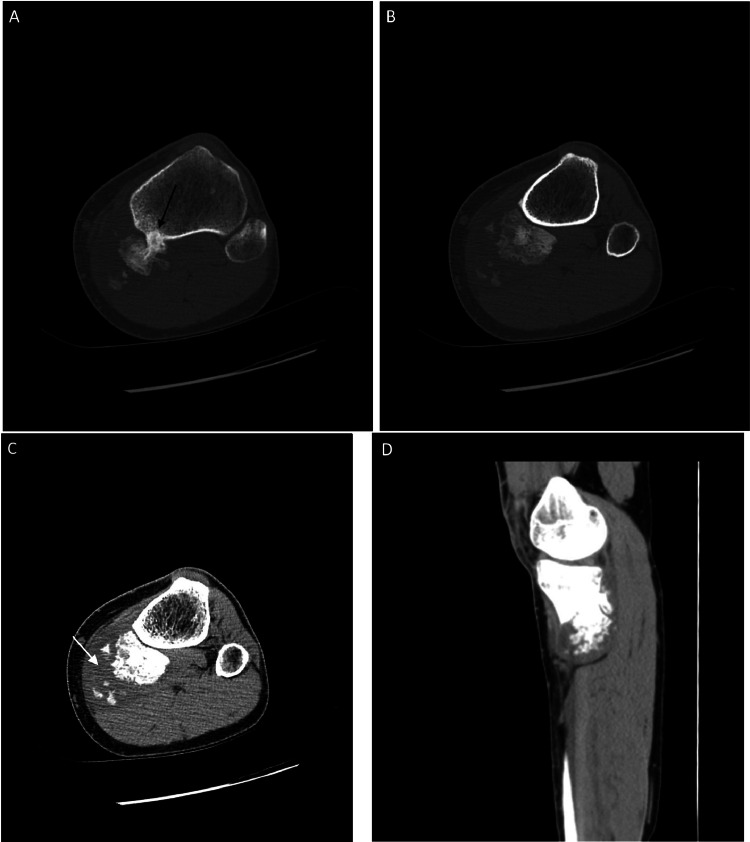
CT of the left tibia. (A and B) Axial bone window images show a parosteal mass at the proximal posteromedial tibia with a small component extending into the medullary space (black arrow) and a central osteoid matrix. (C and D) Axial and sagittal soft tissue window images show the hypodense periphery of the mass (white arrow).
